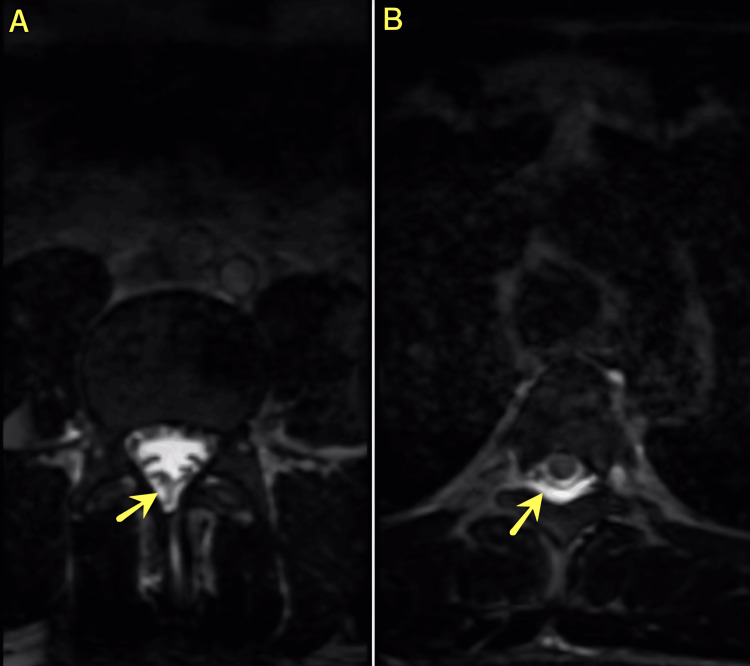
Axial high-resolution T2-weighted MRI demonstrating the dural defect and associated CSF leakage. (A) A small dural defect is visualized along the right dorsal aspect of the lumbar thecal sac at the level of L5-S1. (B) A T2 hyperintense collection is seen along the dorsal aspect of the thoracic thecal sac, consistent with a CSF fluid leak. CSF: cerebrospinal fluid
